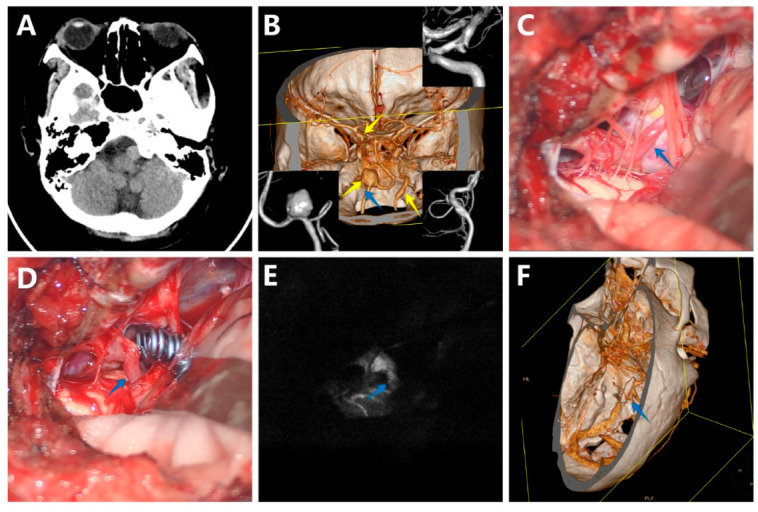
A woman with a large saccular aneurysm on the V4 segment of the right vertebral artery (VA), a small saccular aneurysm on the C7 segment of the left internal carotid artery (ICA), and a fusiform aneurysm located in the V3 segment of the left VA. (A) The CT scan showed a round-like mass located in the front of the medulla oblongata; (B) The digital subtraction angiography (DSA) and CTA disclosed a large saccular aneurysm located in the V4 segment of the right VA, a small saccular aneurysm located in the C7 segment of the left internal carotid artery, and a fusiform aneurysm located in the V3 segment of the left VA; (C) The aneurysm, VA, and posterior inferior cerebellar artery (PICA) before clipping; (D) The aneurysm, VA, and PICA after clipping; (E) Intraoperative indocyanine green videoangiography confirmed no residual aneurysm and patency of VA and PICA; (F) Postoperative CTA further demonstrated complete obliteration of the aneurysm. (The yellow arrows show the aneurysm, and the blue arrows show the PICA).
